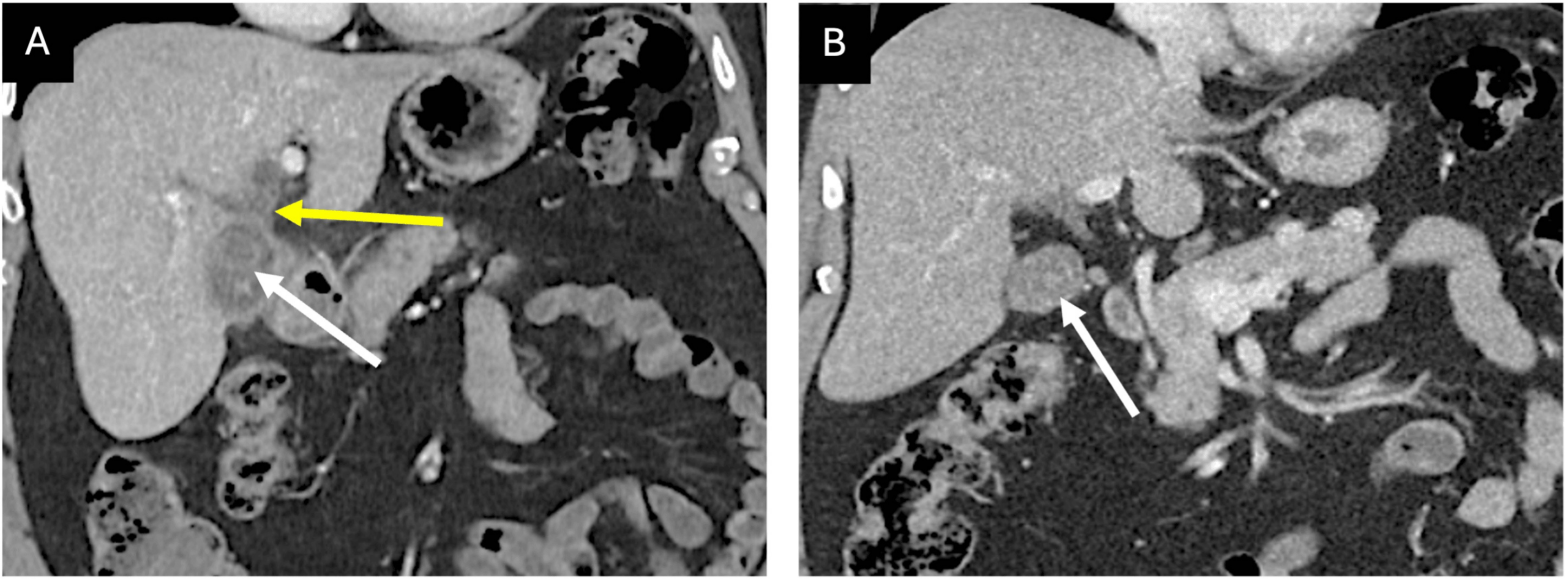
Computed tomography (CT) scan findings at presentation to the outpatient clinic (A) compared to imaging four years prior (B). Portal venous phase CT coronal image (A) demonstrating loss of intervening fat plane between the gallbladder neck and the bile duct in the region of the hepatic duct confluence (yellow arrow). Comparatively, imaging from four years earlier (B) demonstrates a preserved fat plane in the porta between the gallbladder and the common hepatic duct. Gallstones are faintly visible in both scans (white arrows).
